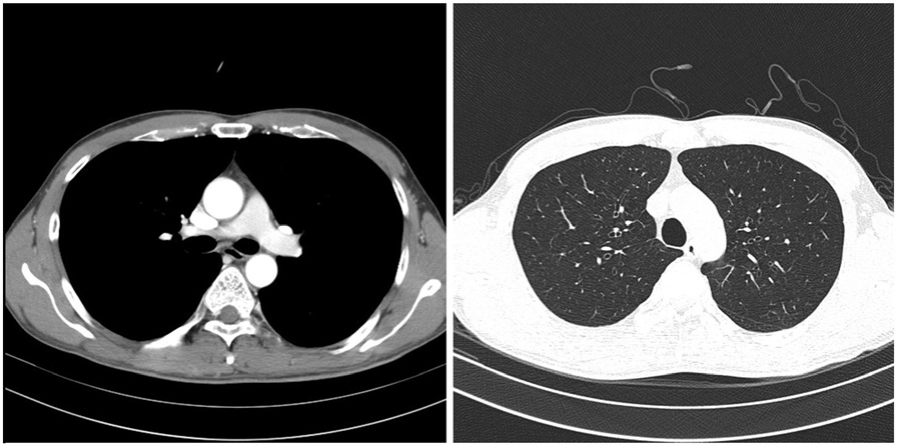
Chest CT findings of case 1 show no abnormality.
